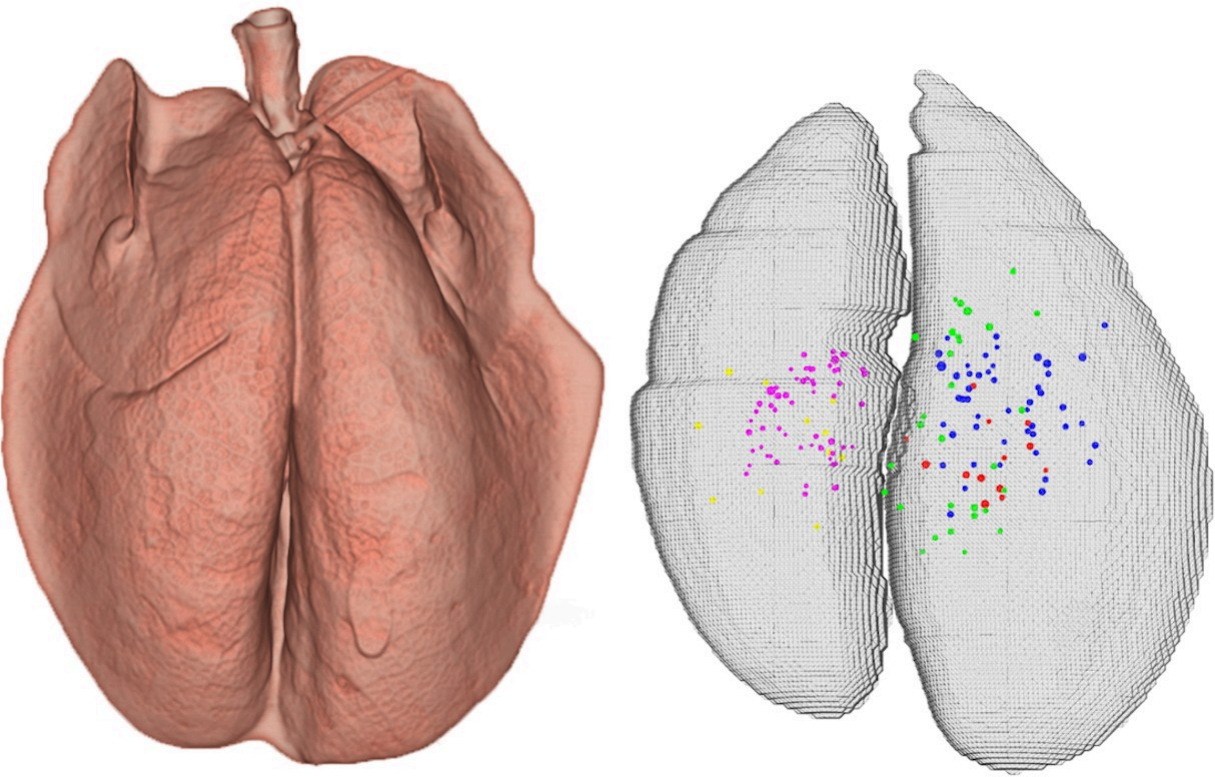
Summary of experimental results. Left: CT image reconstruction of a minipig’s pulmonary surface. Right: 3D representation of location and size of all minipig lesions; each colour is for a different minipig (red, magenta, blue, yellow, and green).
